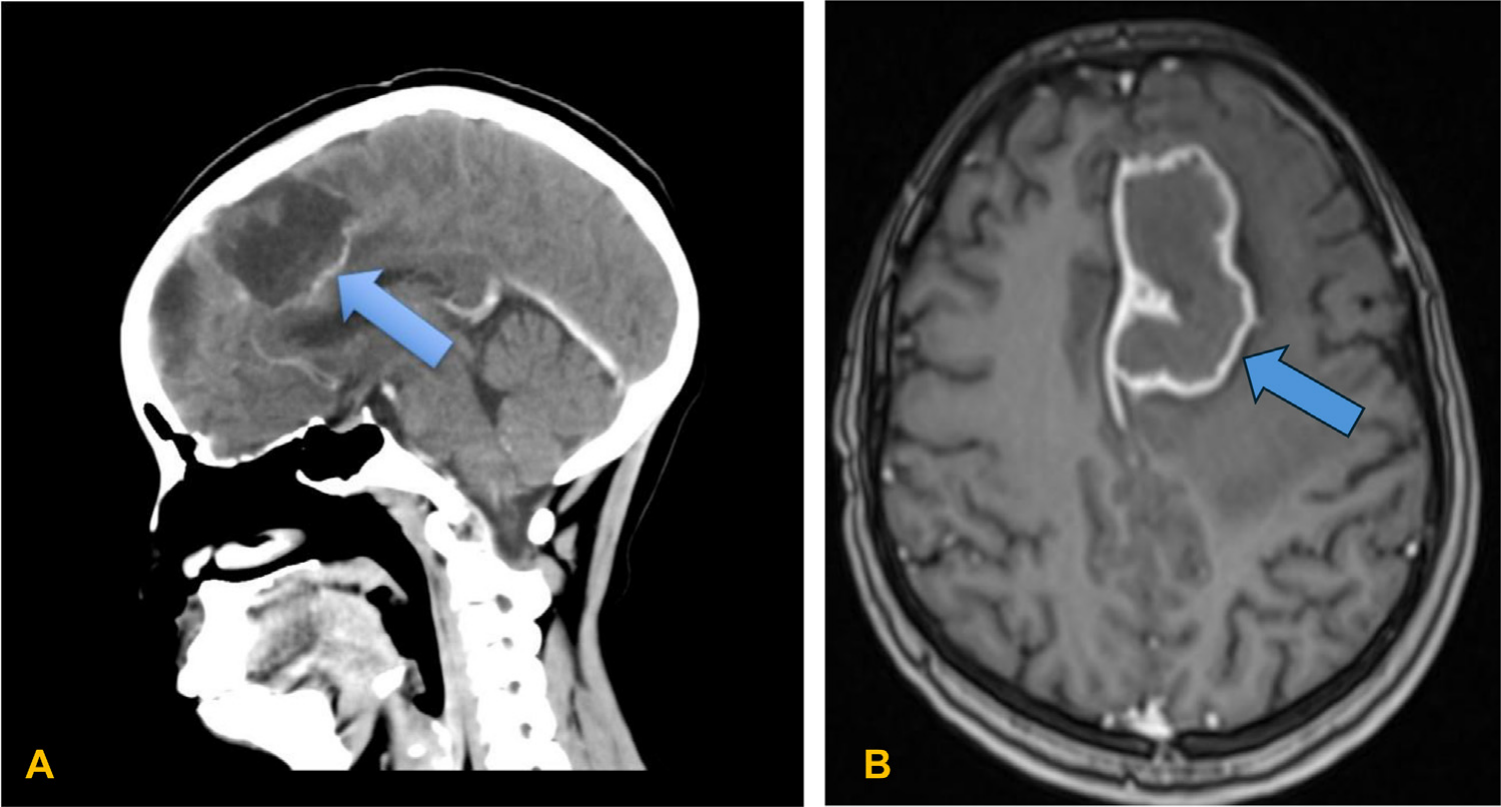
(A) Brain MRI showing a left parafalcine intra-axial mass (blue arrow). (B) Axial T1-weighted post-contrast image demonstrating a heterogeneous ring-enhancing anterior midline mass along the left parafalcine region, with mass effect on the ipsilateral frontal lobe, approximately 1.3 cm midline shift, and subfalcine herniation to the contralateral side.
